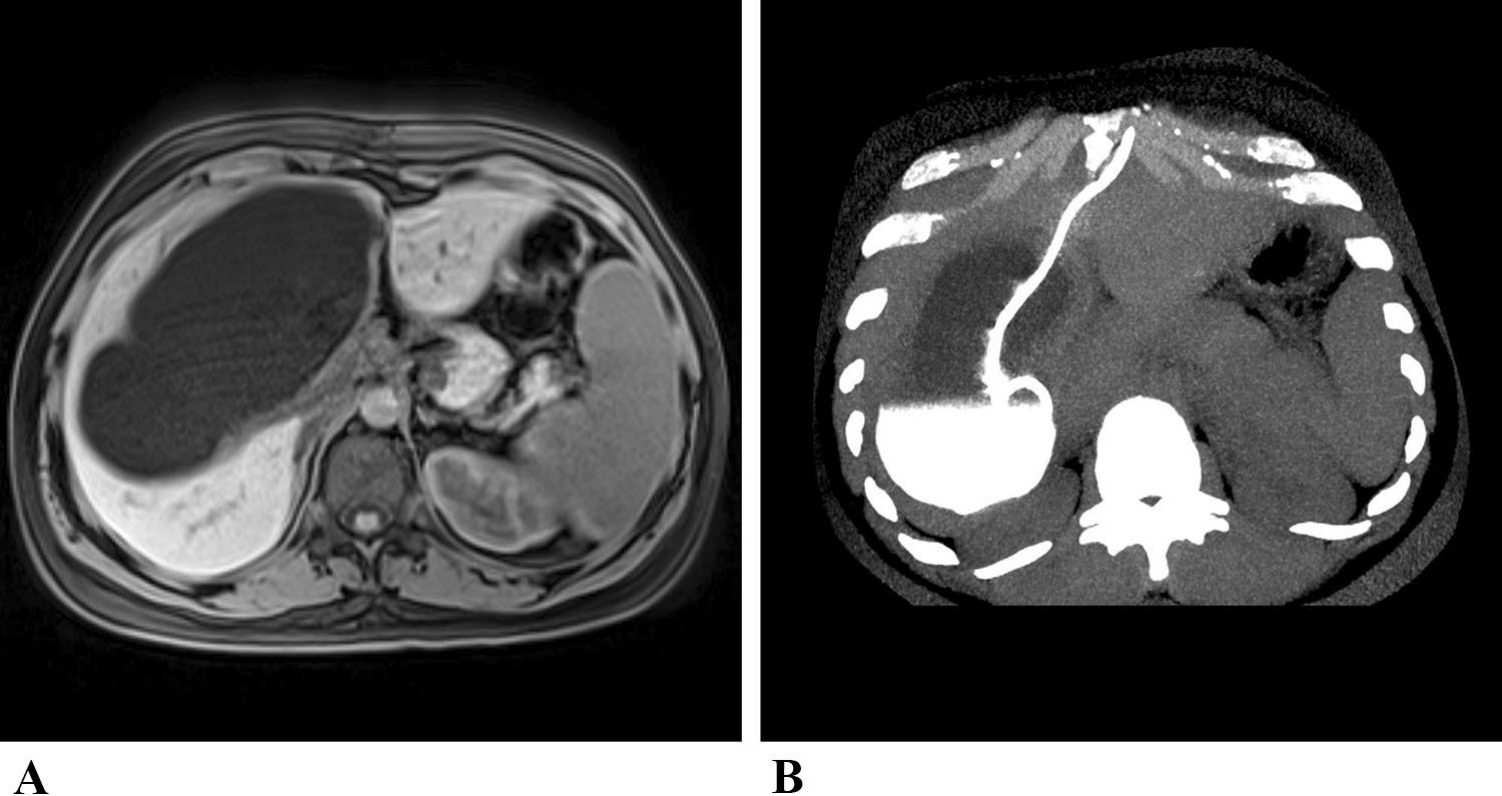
a Magnetic resonance imaging of the giant hepatic echinococcal cyst; b Computed tomography reconstructed in maximum intensity projection (MIP) after catheter placement. The cyst is partially filled with contrast medium
